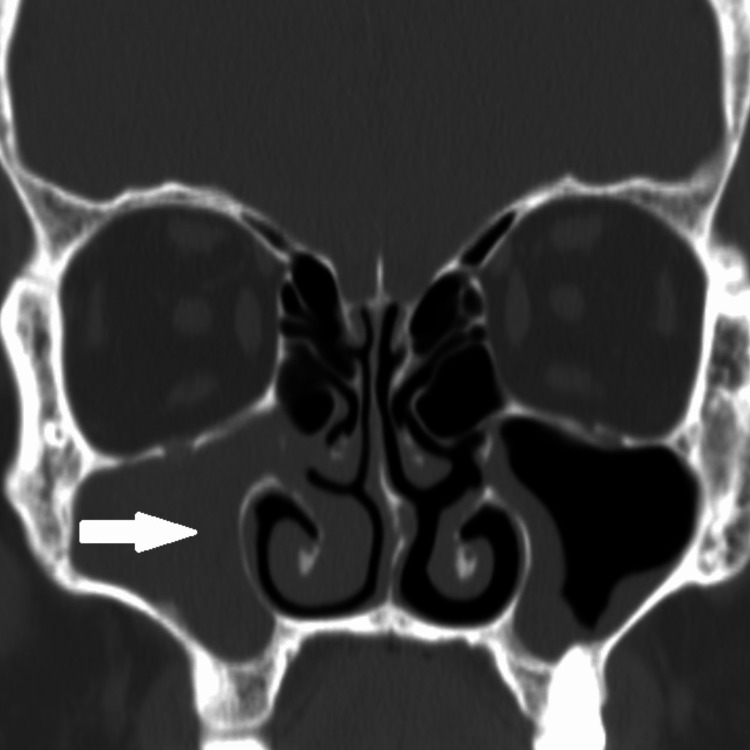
Pre-operative coronal bone window CT image at the level of maxillary sinus showing soft tissue density filling the right maxillary sinus; the bony contours of the sinus remain intact (white arrow).
